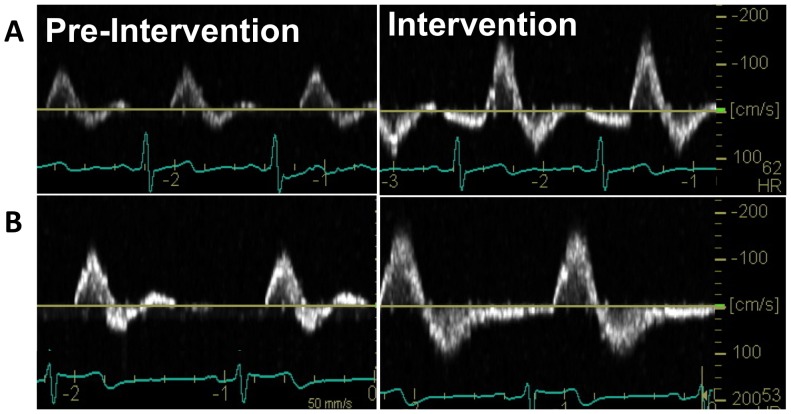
Superficial Femoral Artery Cuff-Inflation Intervention (Doppler Screen Capture). Screen capture of the Doppler velocity profile before and at 30-minute of the (A) 75 mmHg and (B) 100 mmHg cuff inflation in the cuffed leg.
